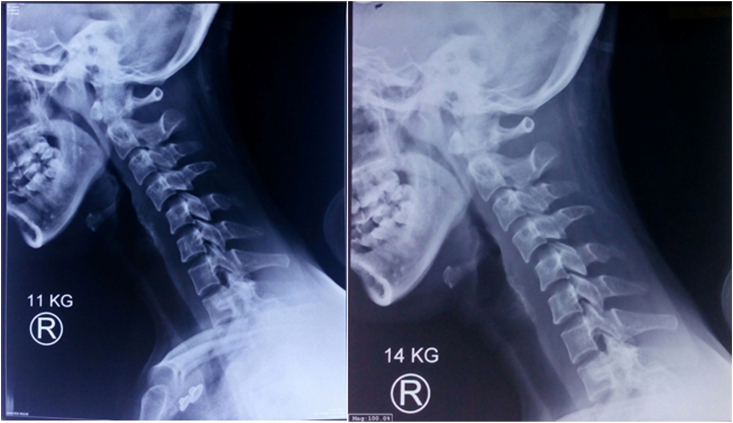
Lateral Radiograph of Cervical Spine.
